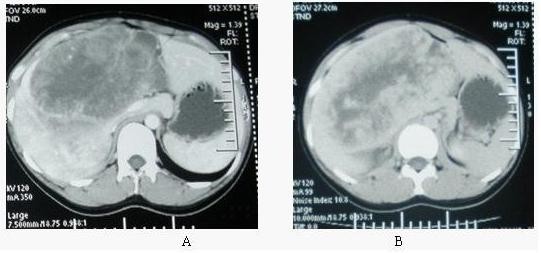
Enhanced CT scan before hepatectomy. A: Arterial phase image showing a large, heterogeneous mass of the liver with hypodense areas, which had clear border between tumor and the normal liver. Lesion showed heterogeneous enhancement and the high density of tumor blood vessels was seen in this region, lower density area to the central region as the center radial arrangement. Left hepatic vein was be compressed by the tumour and turned out to be rigidity. B: Venous late phase images showing a large, solid mass of the liver. Large hypodense tumor of the right hepatic lobe with an enhancement of the peripheral portion. The tumour was diffuse enhanced with a lager hypodense area in the center thought to be necrosis.
